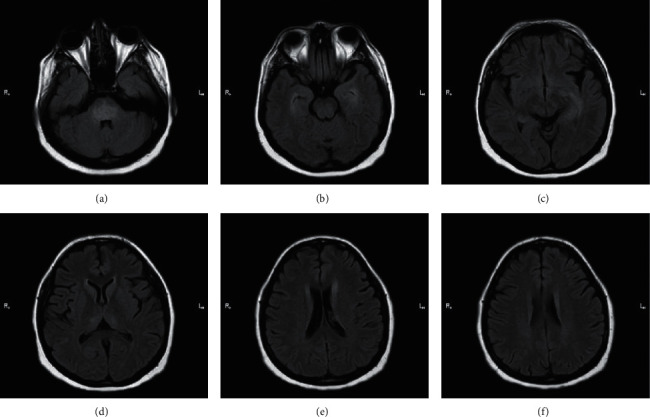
(a–f) Consecutive axial fluid attenuation inversion recovery sequence images of brain MRI without contrast. Increased signal intensity is evident in the pons (a), medial temporal structures (b, c), and thalami (d). Upper corticospinal pathways are spared (e, f).
